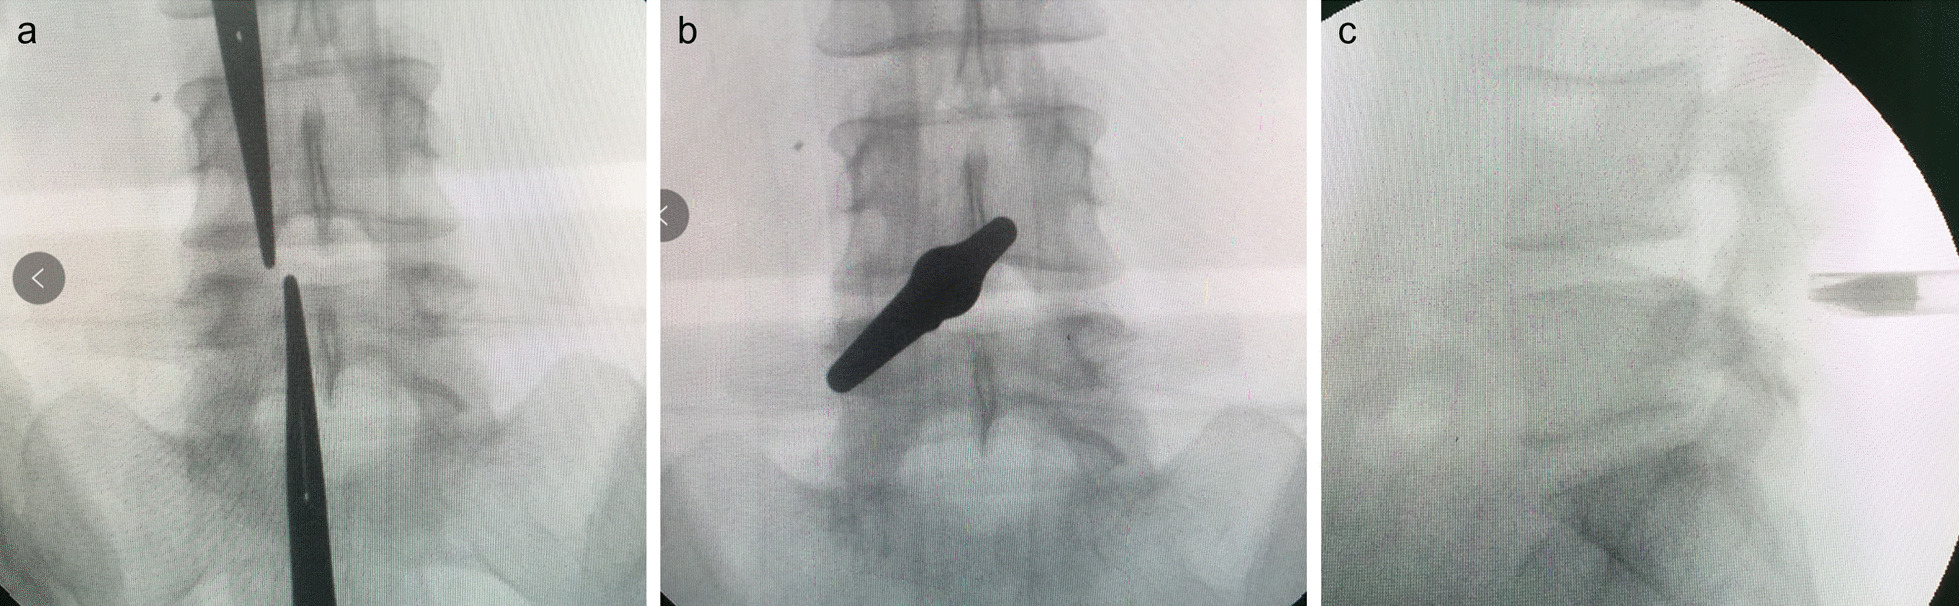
C-arm radiograph during surgery revealing the position of the sleeve. a C-arm was used to locate the lesion stage, and haemostatic forceps were used as reference; b, c Place the dilator
